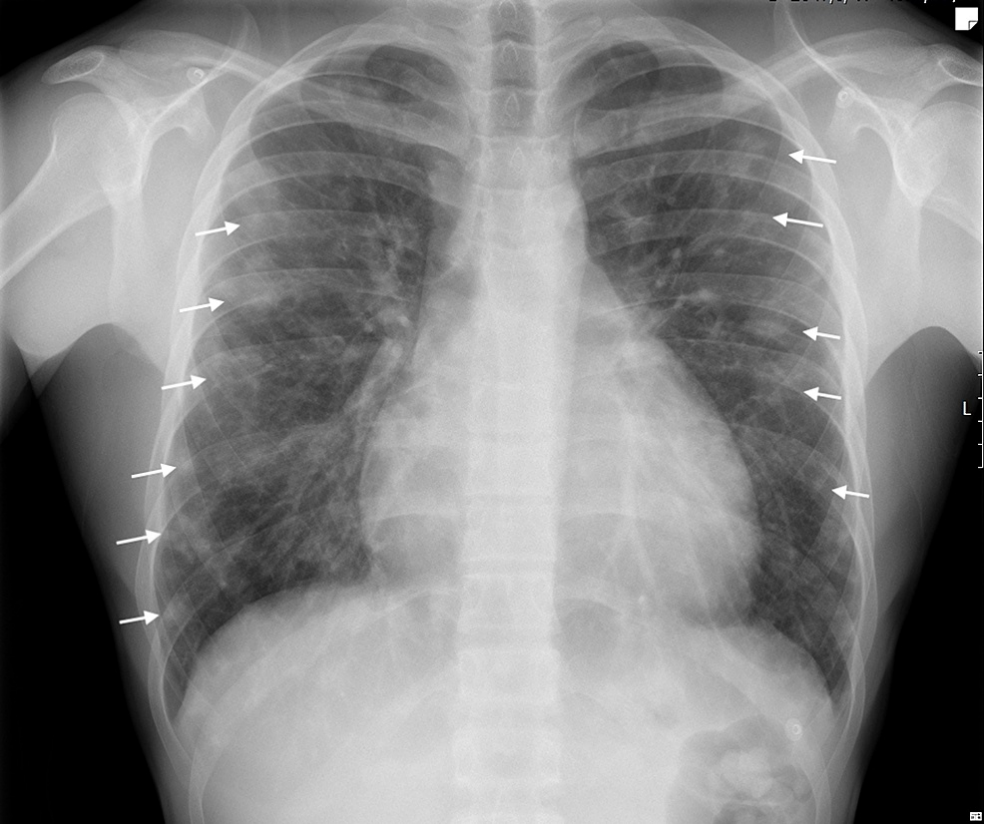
Chest radiograph (posteroanterior view). Multiple, bilateral, nodular opacities of variable size (white arrows) and cardiomegaly.
